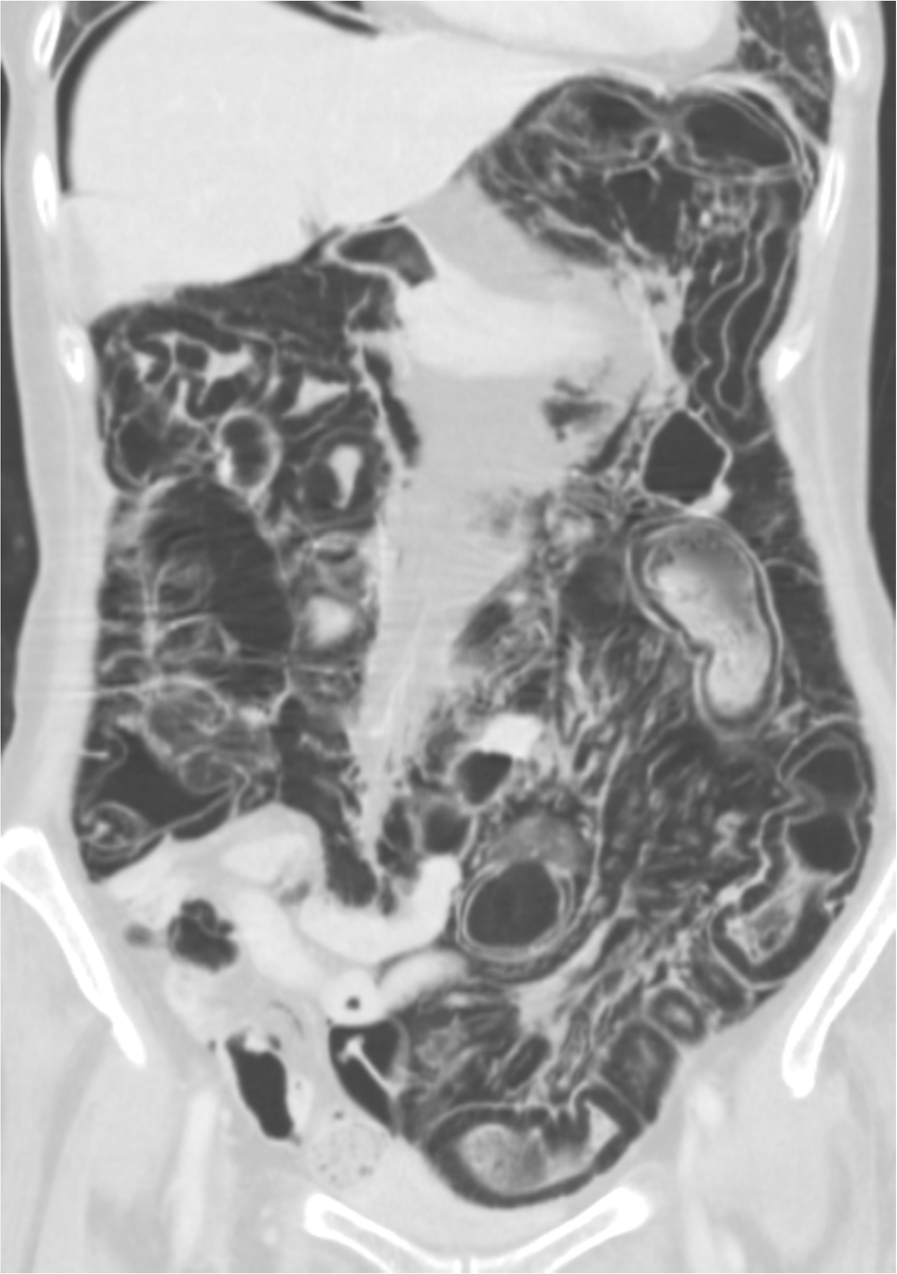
A CT scan of the abdomen demonstrated a thickening of the colon with free air, pneumoretroperitoneum, and pneumomediastinum
